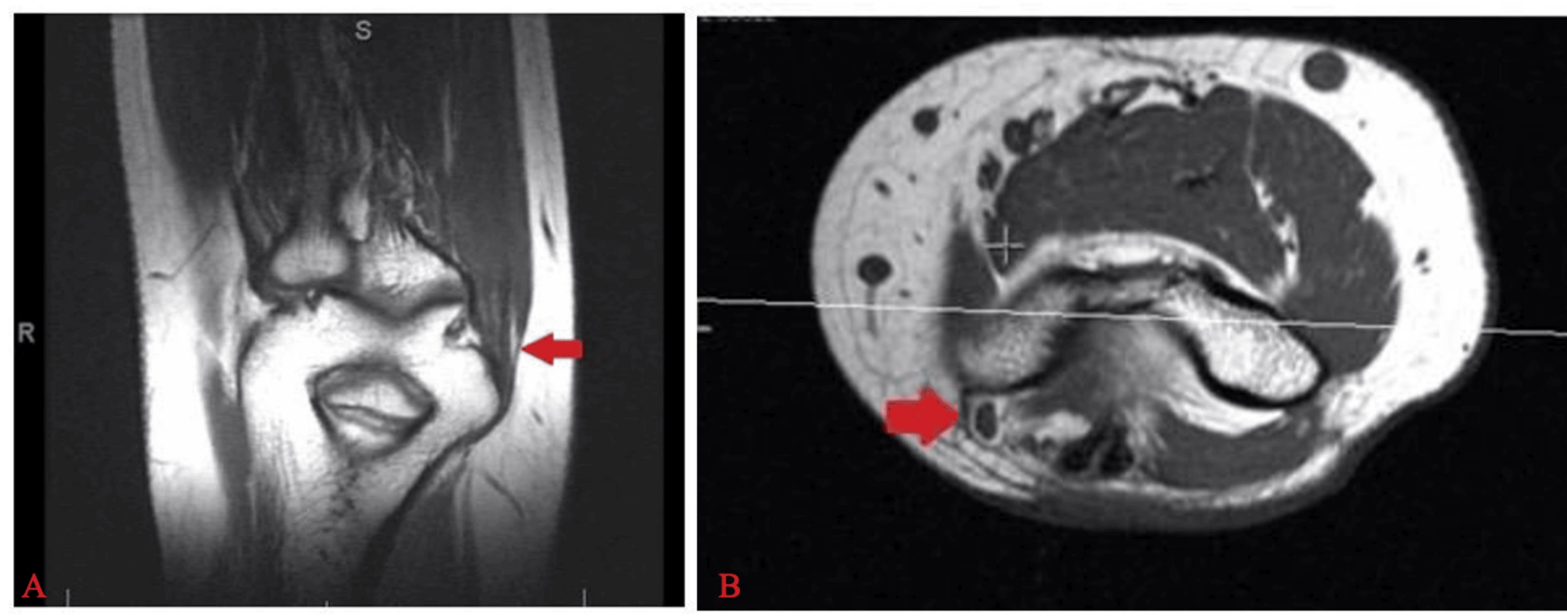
Preoperative ulnar nerve inflammation (A) Coronal T1-weighted MRI of the ulnar nerve, with the red arrow indicating ulnar nerve inflammation. (B) Axial T1-weighted MRI of the ulnar nerve, with the red arrow indicating ulnar nerve inflammation.
